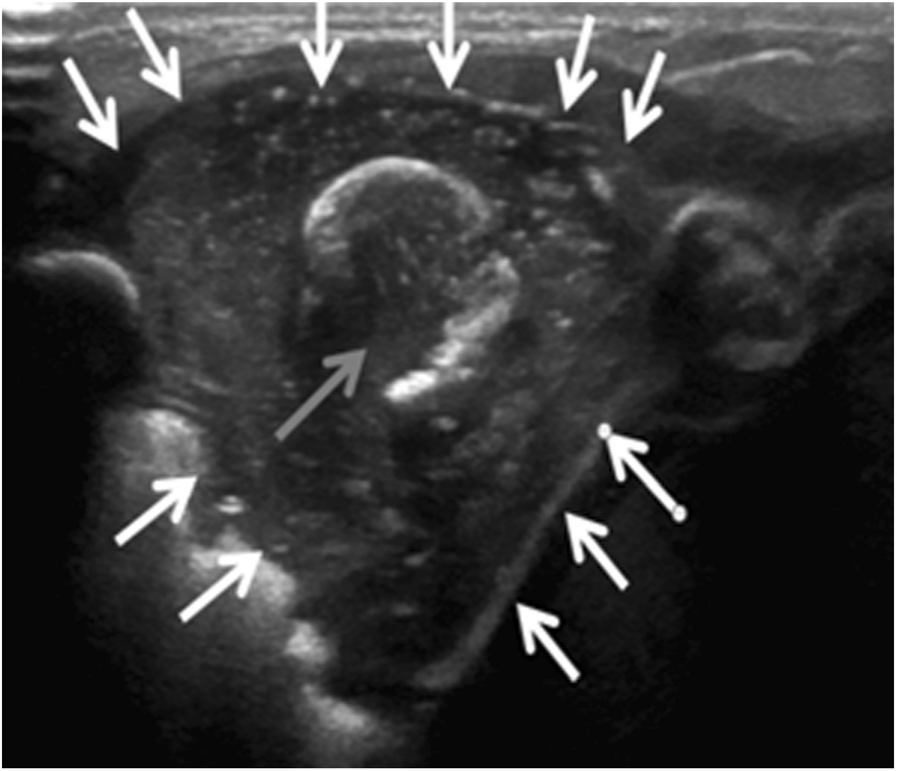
Ultrasonography. There is a hypoechoic fluid collection (white arrows), related to an abscess, encasing a fragmented rib (grey arrow)
